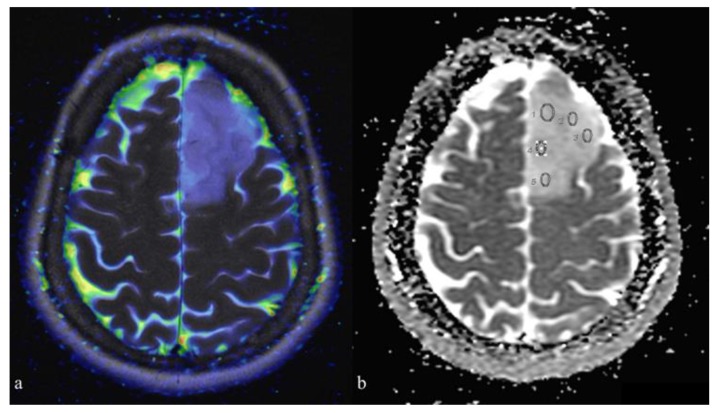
Placement of region on interests (ROIs) on the apparent diffusion coefficient (ADC) map: (a) co-registration between T2-weighted and color-coded ADC map (blue: restricted diffusion; red: facilitated diffusion); (b) placement of five elliptical ROIs on parametric ADC map within the tumor. Co-registration allows identification of the different components of the tumor and peritumoral region. Scale bar: 5 cm.
